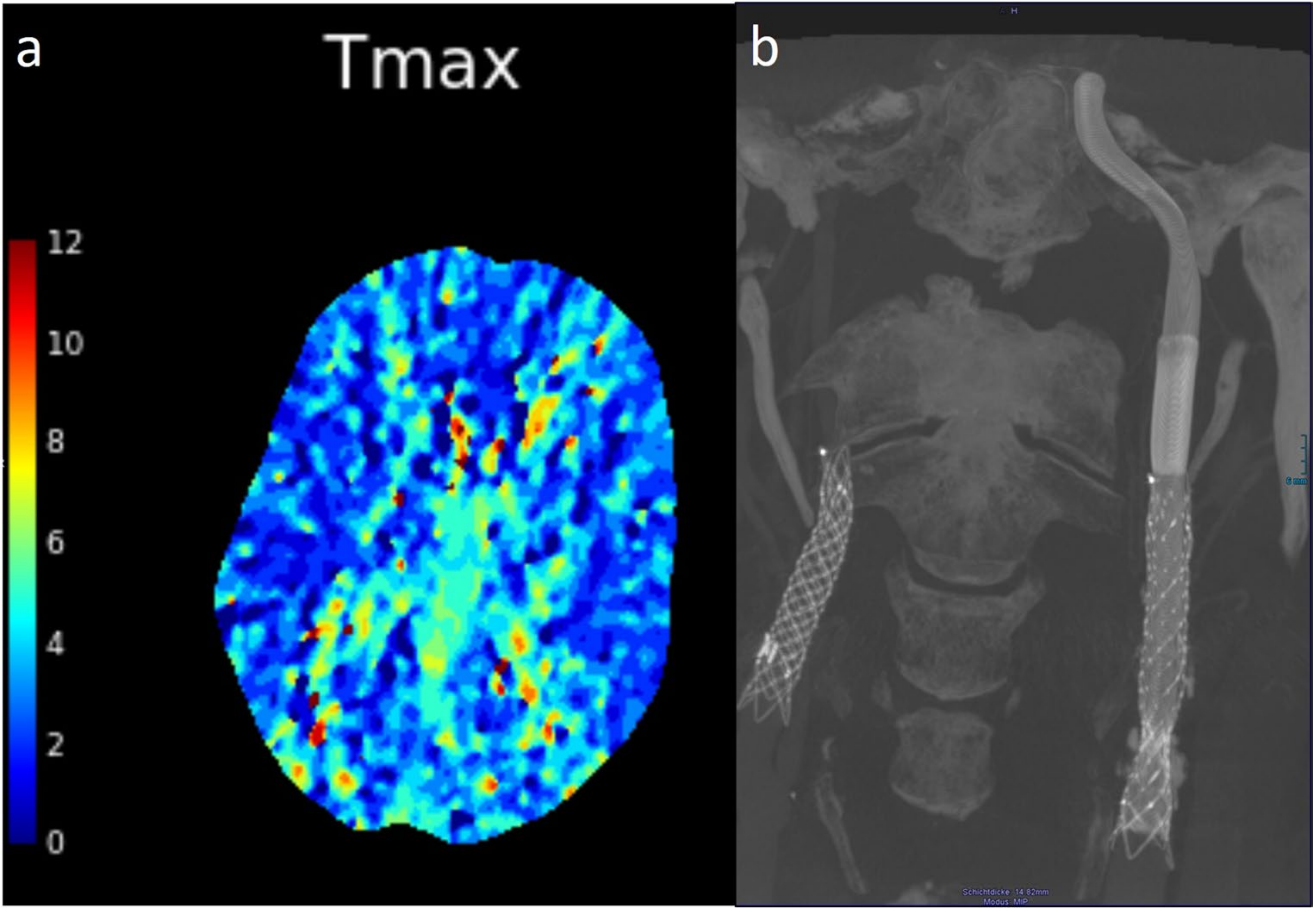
(a) CTP 24 h after stenting showing complete normalization of left hemisphere perfusion. (b) 6w FU with IV conebeam-CT showing free perfusion of the overlapping CARESTOs
